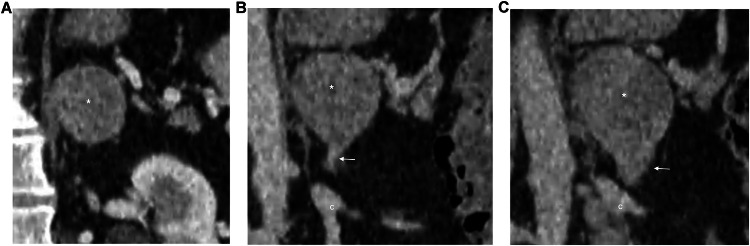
A forty-eight-year-old female had an Invasive Ductal Carcinoma of left Breast resected 6 years ago. All images after intravenous contrast media, in coronal reformation. A A heterogeneous mass (*) is seen on follow-up CT in June. The lesion (*) has a round format. B Abdominal CT, Oct of the same year. The lesion has grown but has changed its format, with an indentation in its inferior aspect. The left adrenal vein (arrow) is seen slightly enlarged, with enhancement similar to other vessels. The left renal vein is seen at a short distance, at the point of the confluence (C) with the gonadal vein. C Mar the following year. The lesion (*) is in continuity with the left adrenal vein (arrow), which is markedly enlarged now, with attenuation identical to the tumor and growing towards the renal vein, at the level of the confluence of the gonadal with renal vein (C)
